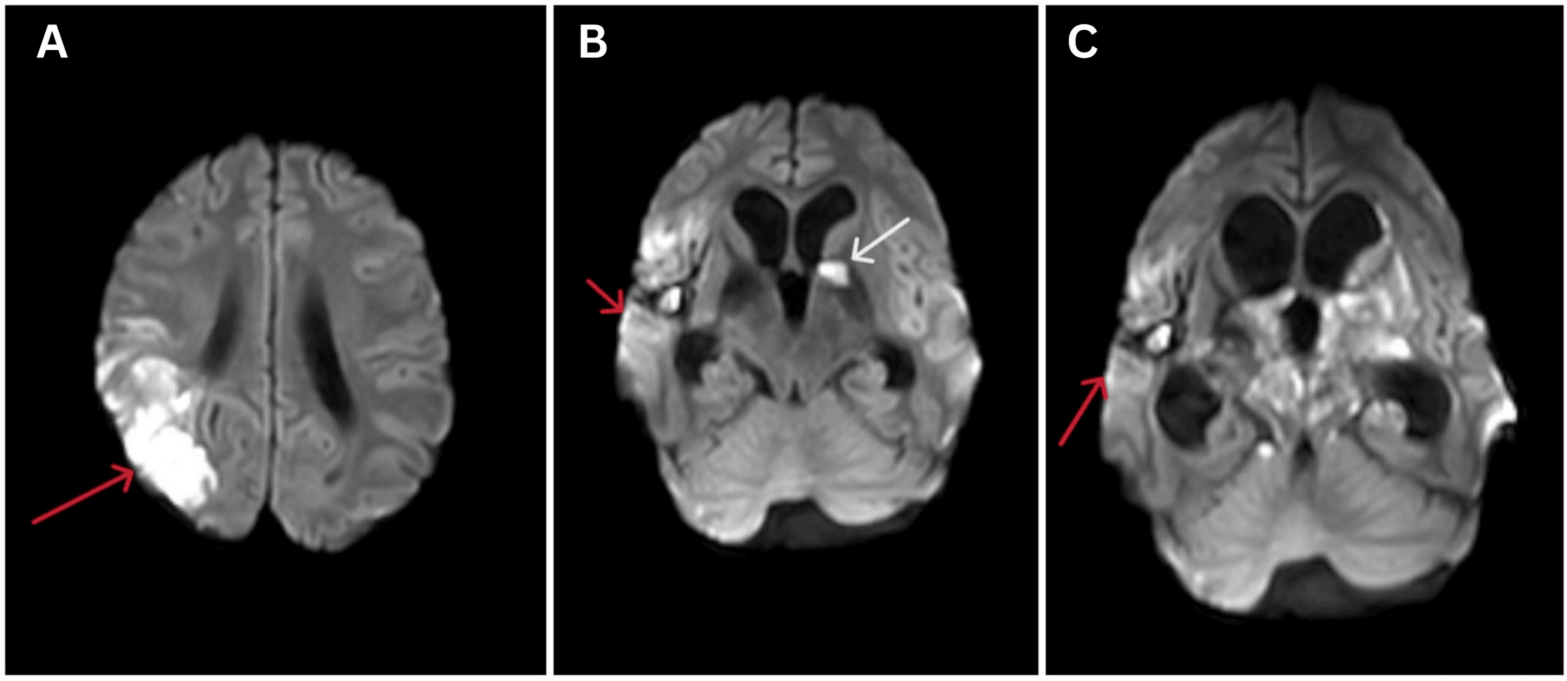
Serial MRI of the brain s/o acute infarcts (A) in the right parietal lobe (red arrow), (B) in the head of the left caudate nucleus, globus pallidus, and left internal capsule (red arrow) with periventricular ooze (white arrow), and (C) in the left lentiform, medial hypothalami, and midbrain (red arrow). MRI: magnetic resonance imaging
